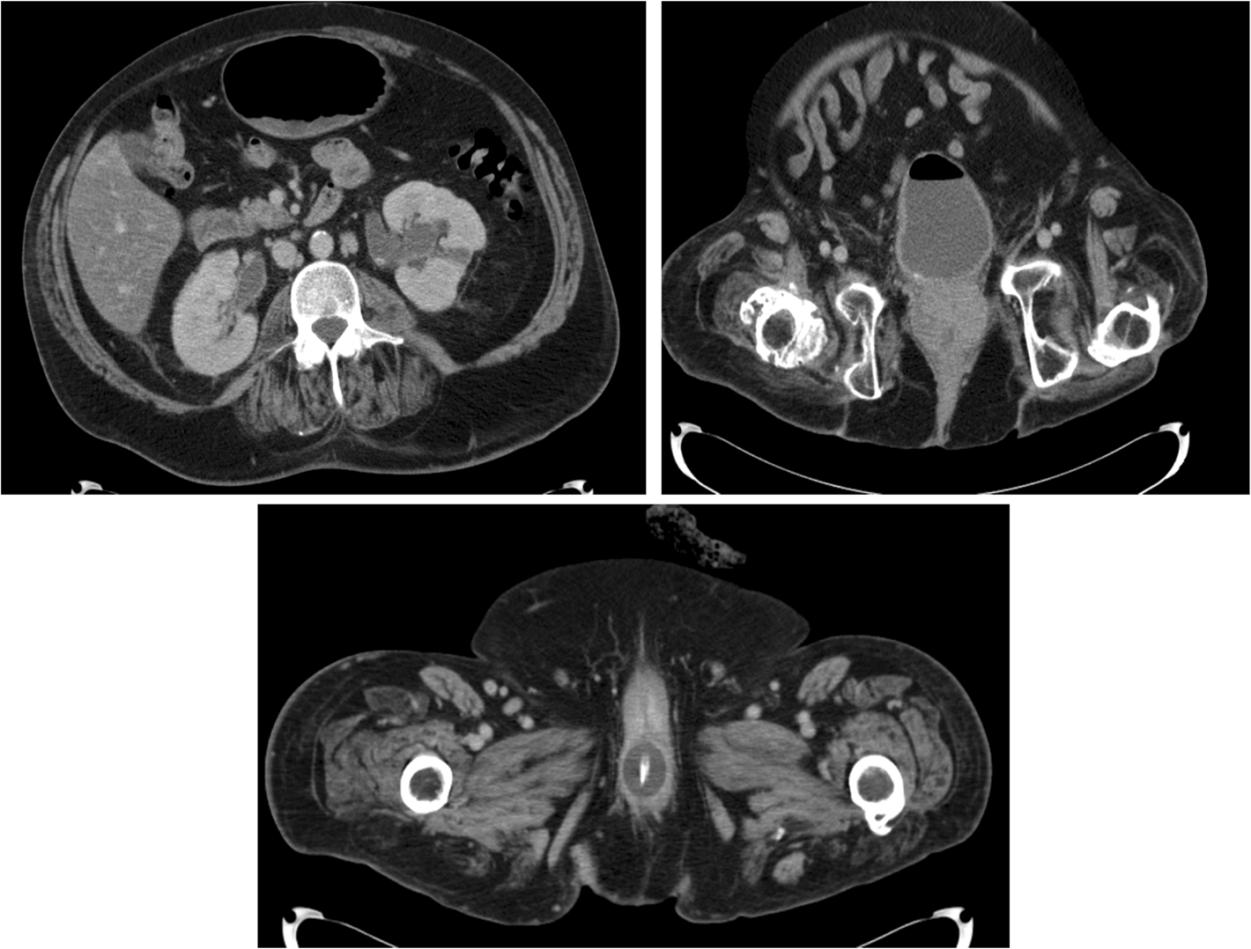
Case 5. Top Left panel: CT abdomen axial section revealed moderate left hydronephrosis and hydroureter, and mild right hydronephrosis and hydroureter. Top Right panel: CT of abdomen and pelvis, axial section: Urethral catheter was not present within the bladder. Bladder was of small capacity and contained urine and gas. Bottom panel: CT pelvis, axial section revealed balloon of Foley catheter in the membranous urethra
